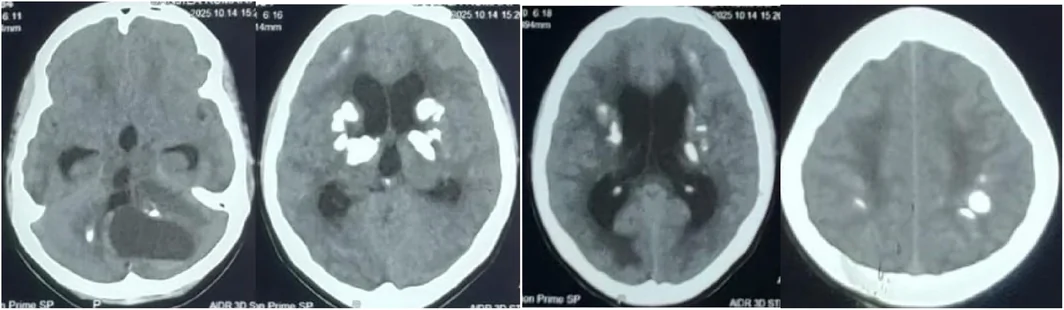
Pre‐op CT Scan of brain showing extensive bilateral basal ganglia, thalami, and cerebellar calcification and calcified cystic left cerebellar mass with hydrocephalus.
